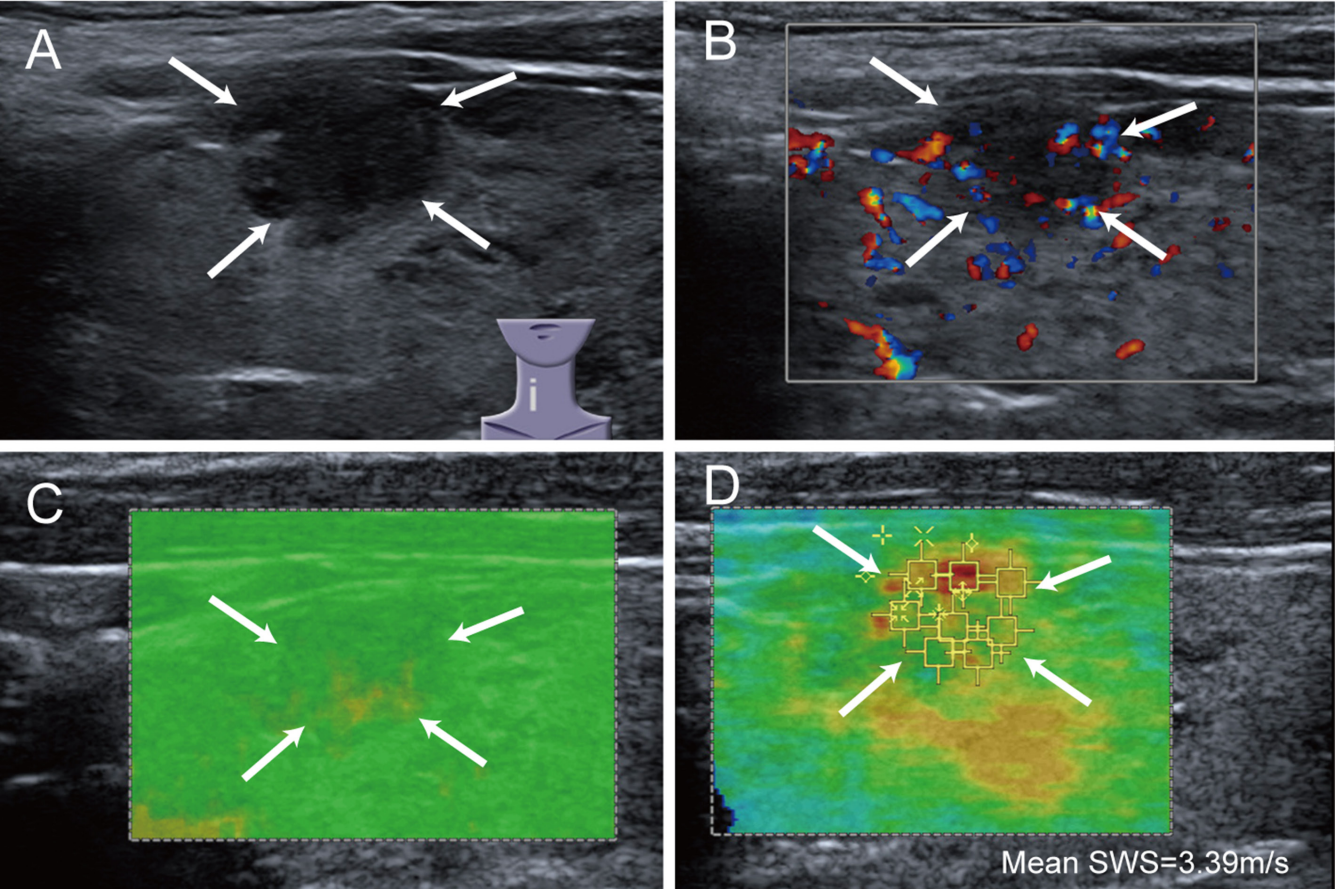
Images of low QM in malignant thyroid nodule A 39-year-old woman has papillary thyroid carcinoma. (A) conventional ultrasound shows a 12 mm thyroid nodule (arrows) in right thyroid lobe, which is solid, markedly hypoechoic and irregular; (B) color Doppler ultrasound shows sporadic color flow signals in the nodule (arrows); (C) SW-quality map shows that internal yellow portions are more than 20 percent of the nodule (arrows), indicating low QM; (D) the mean SWS of the nodule (arrows) is 3.39 m/s.
